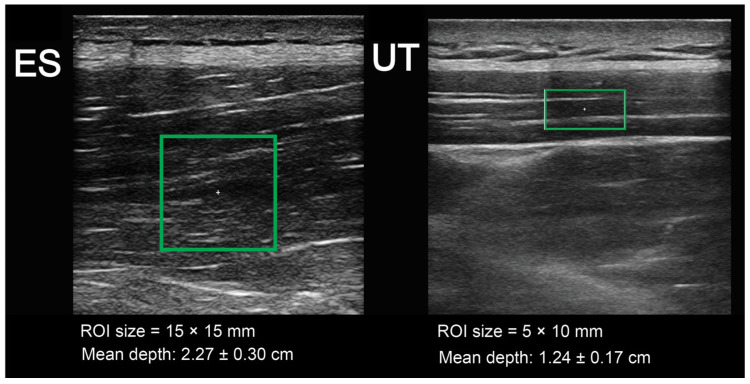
Screenshots of the ultrasound measurements with the regions of interest shown on the muscle tissues. ES, erector spinae; UT, upper trapezius.
